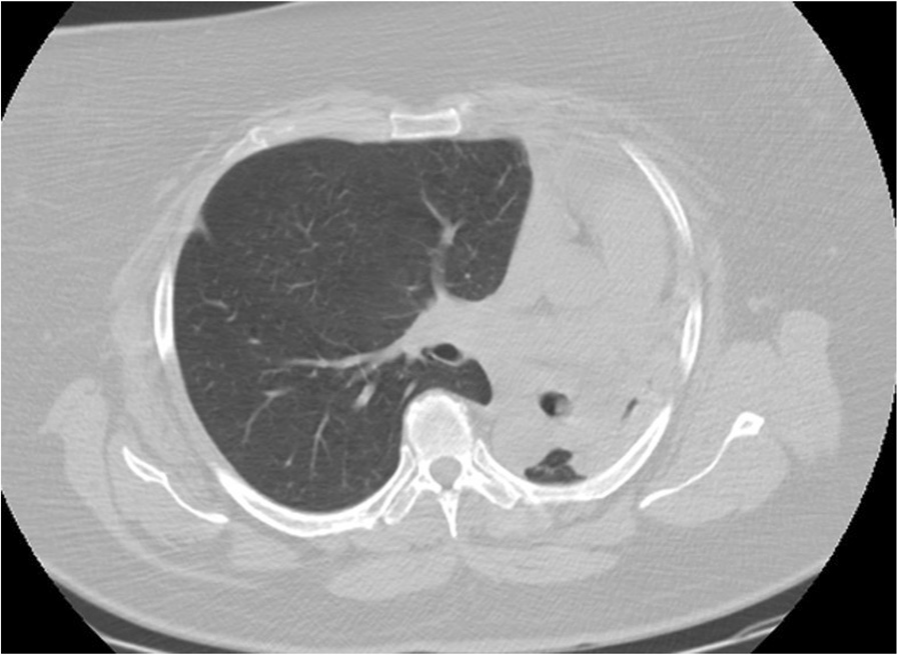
Chest CT image of total atelectasis of the left lung.
